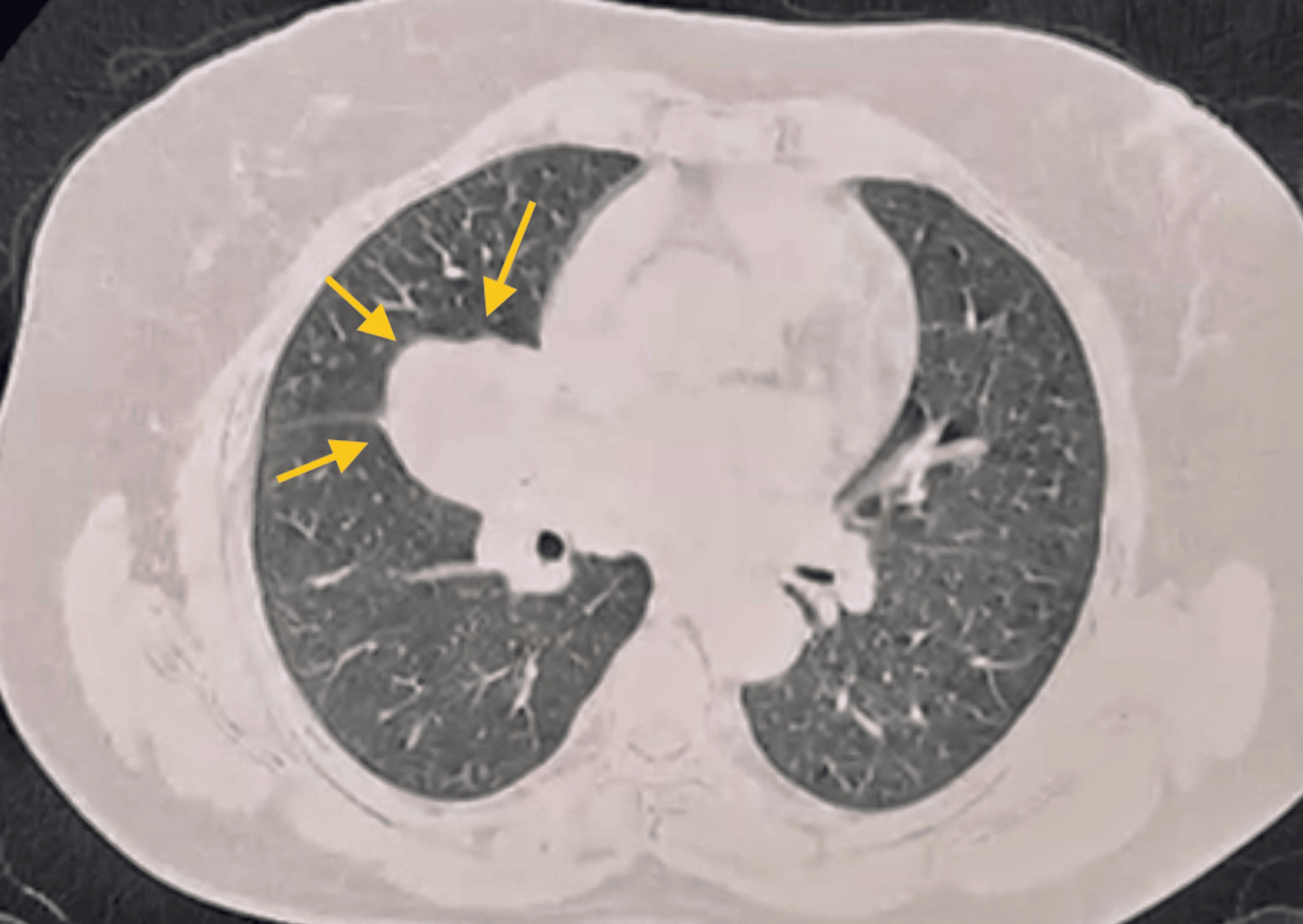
CT of the chest showed a large, well-defined, lobulated soft tissue density lesion (yellow arrows) in the right parahilar region, involving the right middle lobe and superior segment of the right lower lobe
